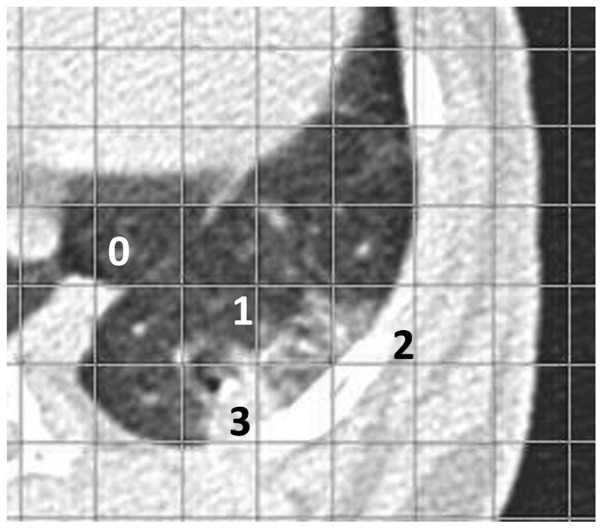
Areas with abnormal density on the computed tomography images. Consolidation, homogeneous ground-glass opacity and reticulolinear shadows were scored as a ratio of the grid as follows: 0, normal; 1, abnormal area <1/4; 2, abnormal area ≥1/4 but <1/2; and 3, abnormal area ≥1/2.
